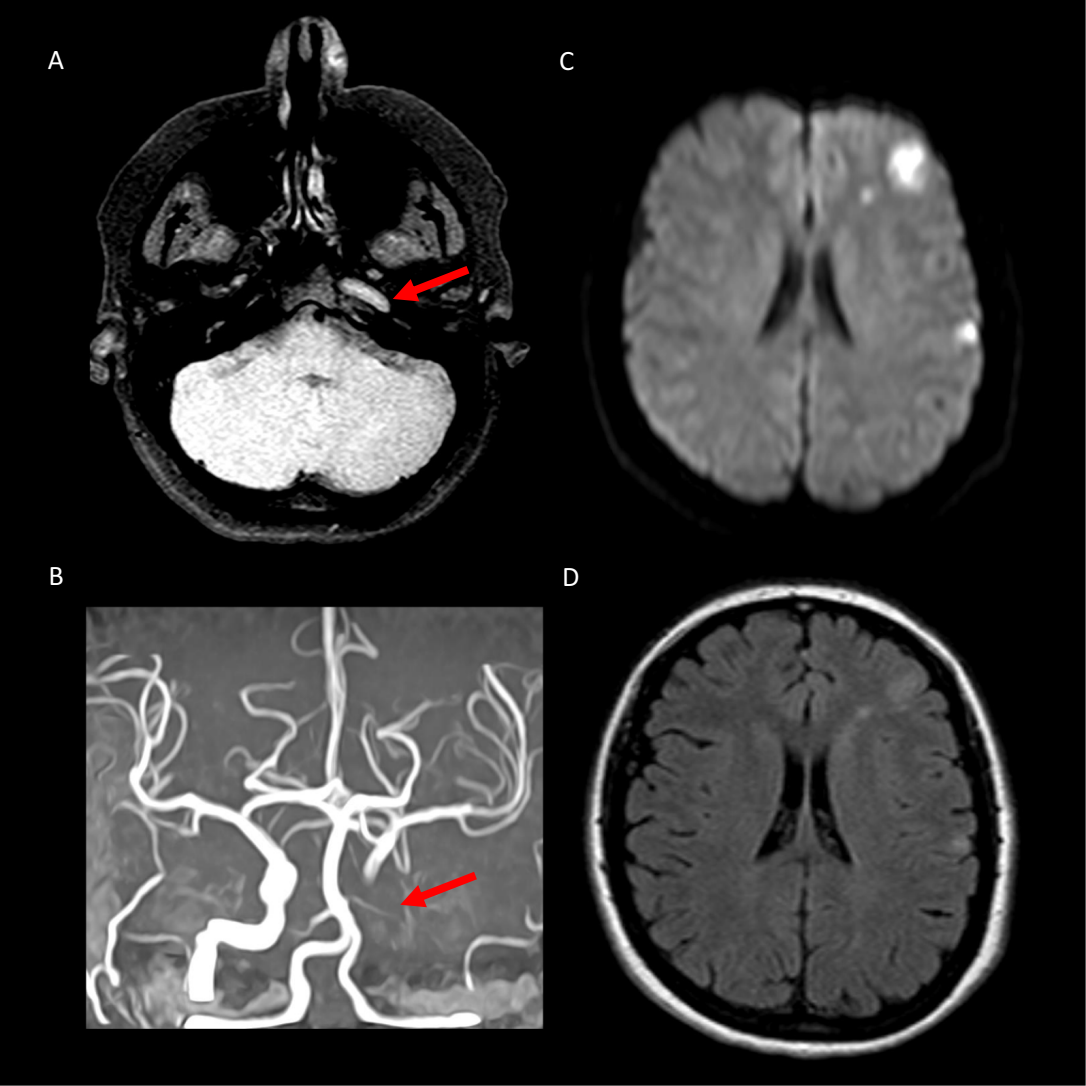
a Axial T1 fat saturated and b coronal Time Of Flight (TOF) MRI images at 1.5 T show evidence of left intracranial ICA thrombotic occlusion in petrous to clinoid ICA segments. c Axial diffusion weighted (DW) and d Fluid Attenuated Inversion Recovery (FLAIR) images reveal concomitant downstream infarction in MCA territory
